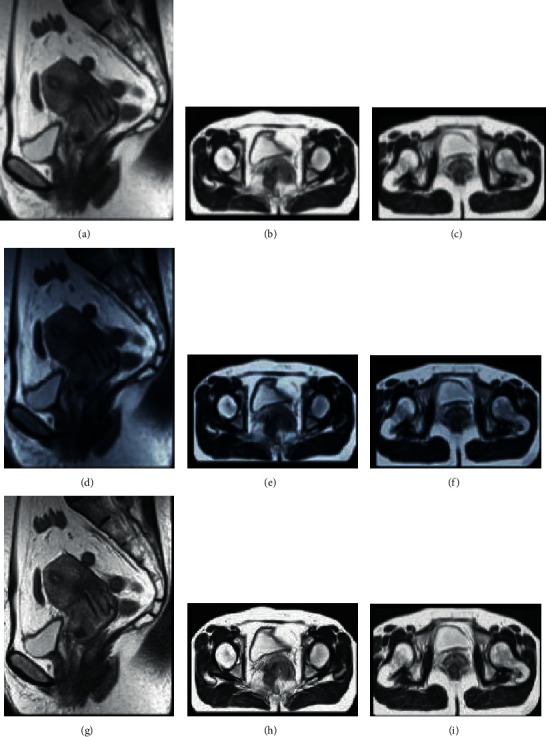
MRI images processed by different algorithms. (a) T2W1 image of normal abdominal MRI sagittal position. (b) T1W1 image of normal abdominal MRI transverse position. (c) T2W1 image of normal abdominal MRI transverse position. (d) T2W1 image of abdominal MRI sagittal position of traditional CNN. (e) T1W1 image of abdominal MRI transverse position of traditional CNN. (f) T2W1 image of abdominal MRI transverse position of traditional CNN. (g) T2W1 image of abdominal MRI sagittal position of artificial intelligence 3D-CNN algorithm. (h) T1W1 image of abdominal MRI transverse position of artificial intelligence 3D-CNN algorithm. (i) T2W1 image of abdominal MRI transverse position of artificial intelligence 3D-CNN algorithm.
